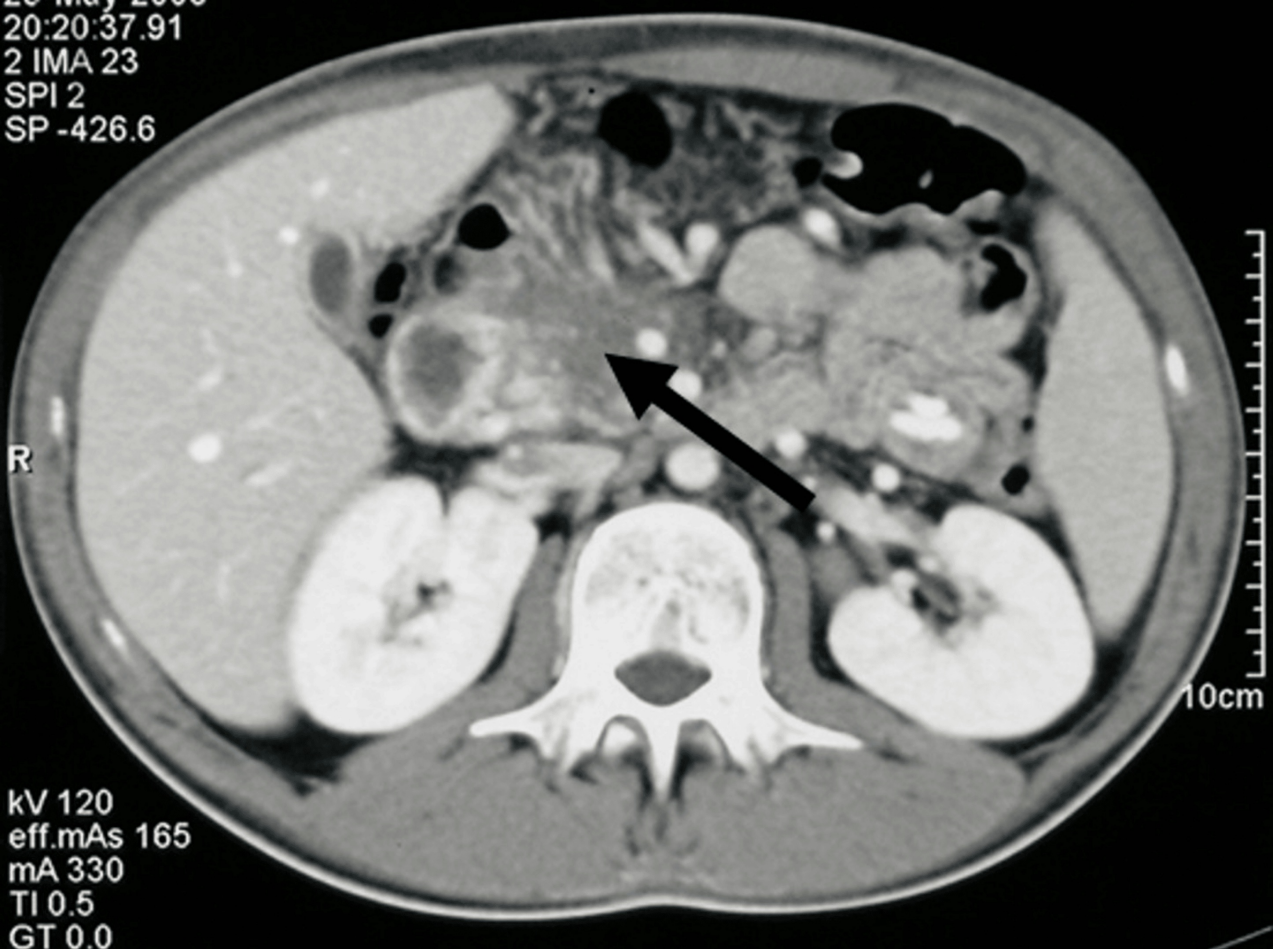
Abdominal computed tomography scan four months after allogeneic stem cell transplantation, demonstrating >90% reduction in pancreatic tumor size (arrow), consistent with a possible graft-versus-leukemia effect.
